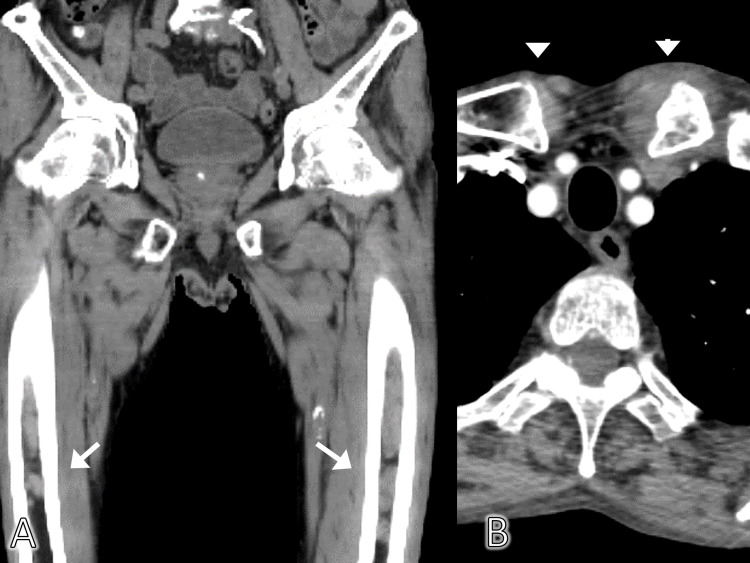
Contrast-enhanced computed tomography of the chest and computed tomography of the legs Computed tomography findings of the leg showed occupied lesions in the bone marrow of the bilateral femur and pelvis (A) with white arrows Contrast-enhanced computed tomography of the chest showing bilateral sternoclavicular joints (B) with white arrowheads
